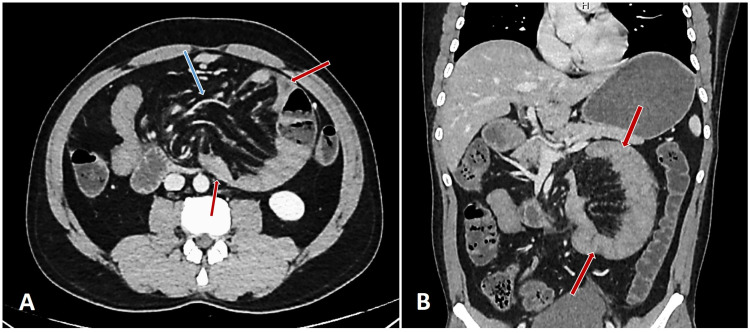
Contrast-enhanced computed tomography (CECT) findings Axial (A) and coronal (B) views of CECT showing typical findings, left paraduodenal hernia (LPDH) namely herniation of bowel loops through an orifice  (blue arrow) and clustered small bowel loops to the left upper quadrant in the anterior pararenal space forming a C- shaped closed loop (red arrows).
